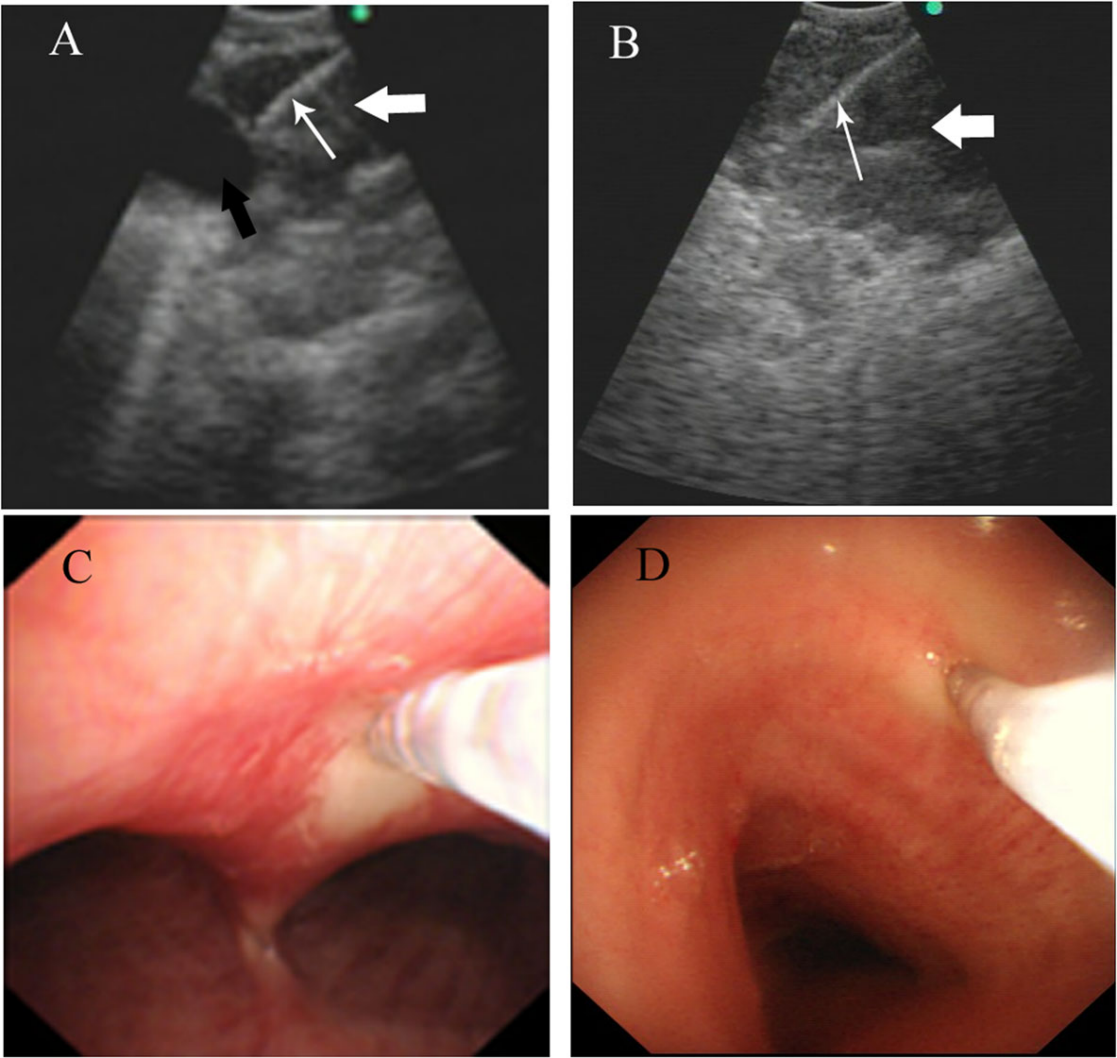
Endobronchial ultrasound-guided TBNA (EBUSTBNA). a The ultrasound image of lymph node biopsy. The needle (*white* slender arrow) was carefully inserted through bronchial wall to the enlarged lymph node (*white* thick arrow), avoiding injuring blood vessel (*black* arrow). b The fine needle (*slender* arrow) was inserted to enlarged lymph node (thick arrow). c and d are transbronchial needle aspiration (TBNA)
